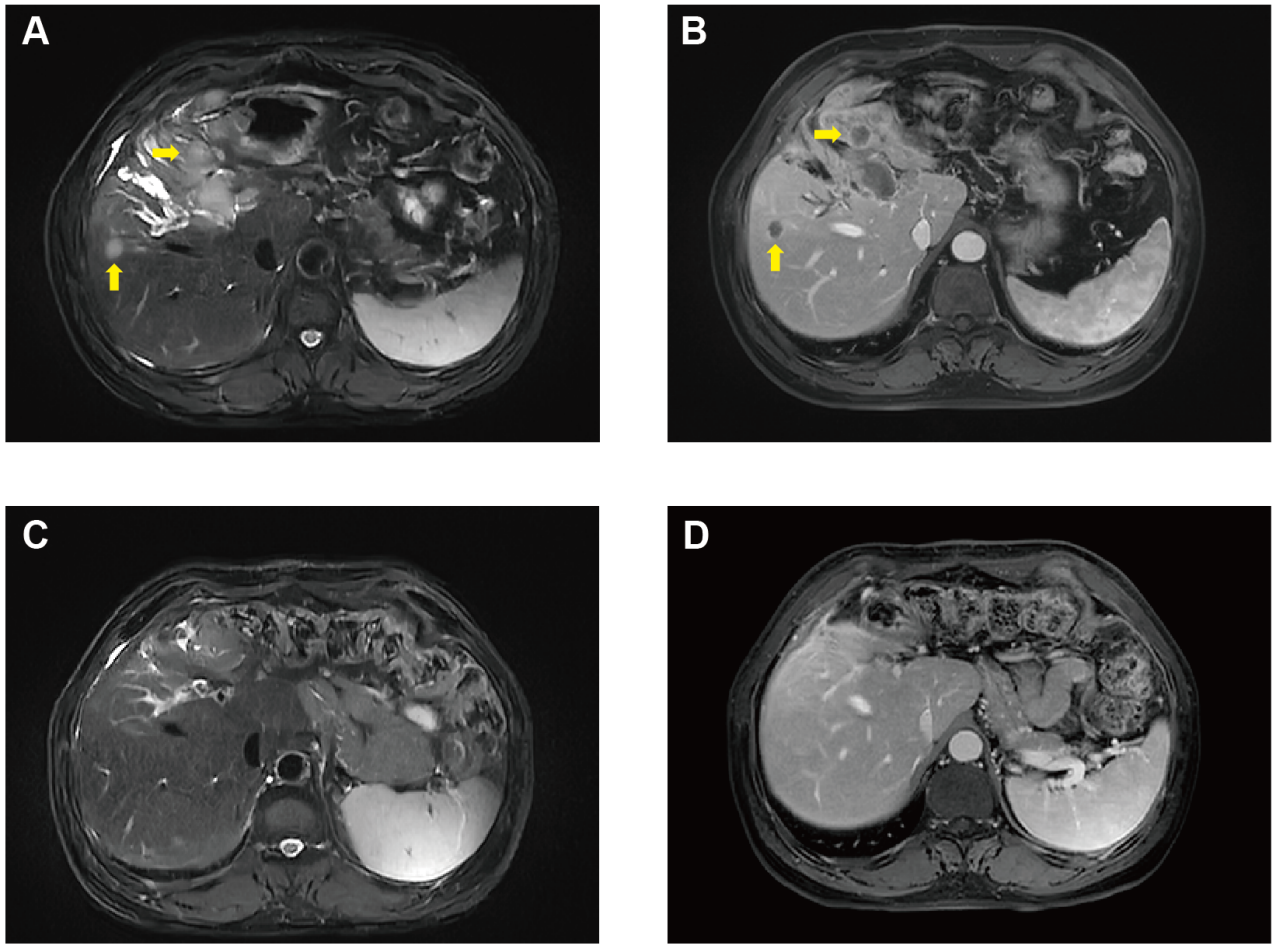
MRI exam changes before (A, B) and after (C, D) ICI therapy.
